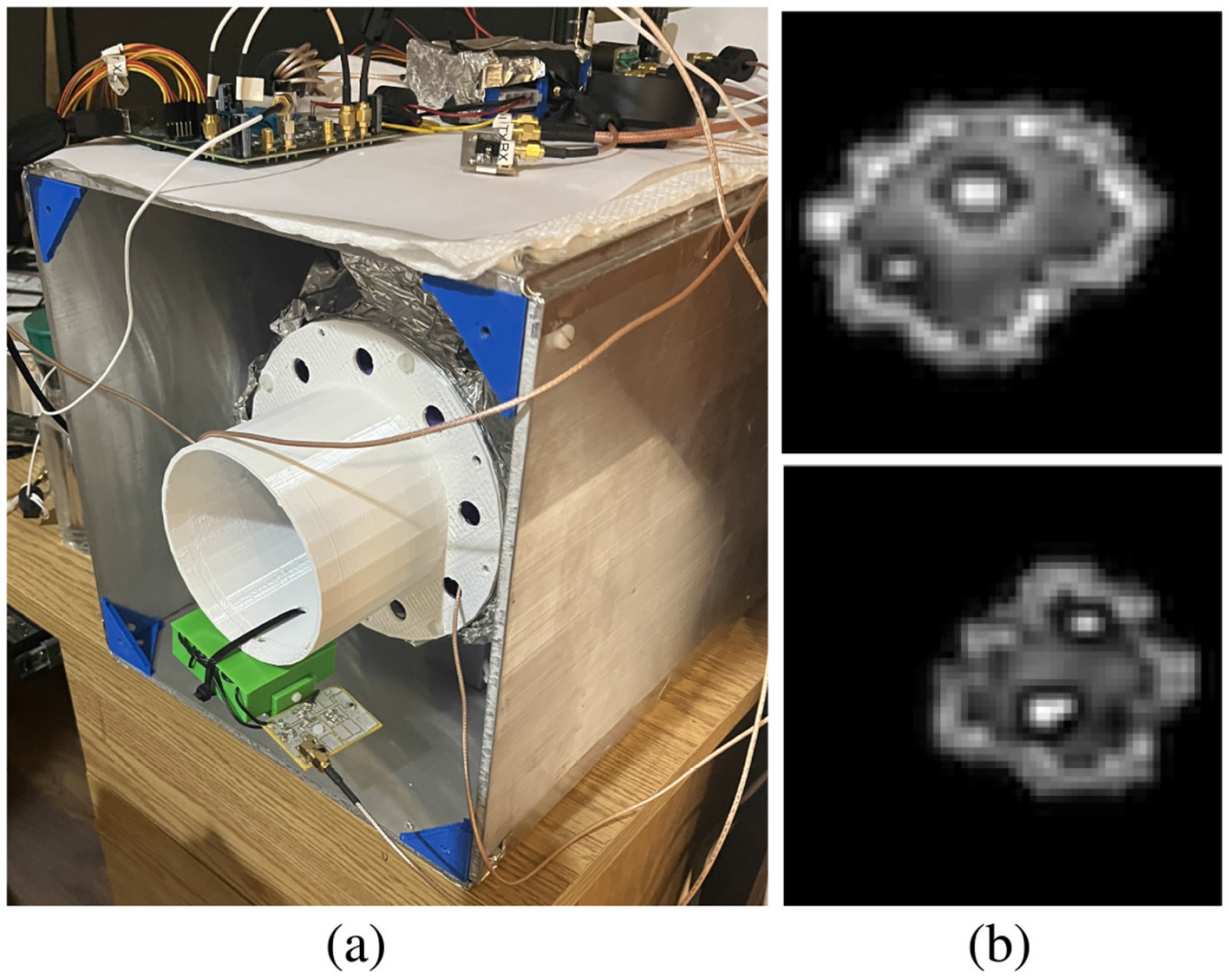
(A) The *LT Imaging Owl MRI*. It has very little in the way of packaging, giving ample opportunity for student-led design solutions for the *SIIT Owl MRI* build. Seen at the bottom of the picture is a test setup for the LNA/tuning board for EDITER coils (Srinivas et al., 2022) that were incorporated into the *SIIT Owl MRI*. (B) Wrist images made with the *LT Imaging Owl MRI* clearly show muscle, skin and the marrow and cortex of the Ulna and Radius bones. An unanticipated axial *B*_1_ phase twist in the thin saddle coil prevents further improvement of these images. Work is ongoing to redesign that coil to achieve a robust 1.5D TRASE process.
